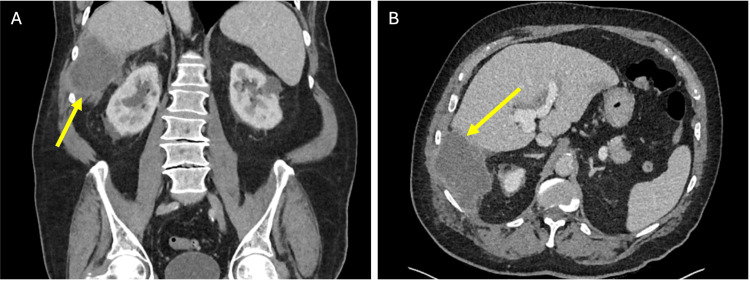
Computed tomography (CT) scan on presentation to the emergency department six months postoperatively with abdominal pain and fevers. Portal venous phase CT demonstrates a new perihepatic abscess (yellow arrows) in coronal (A) and axial (B) views.
